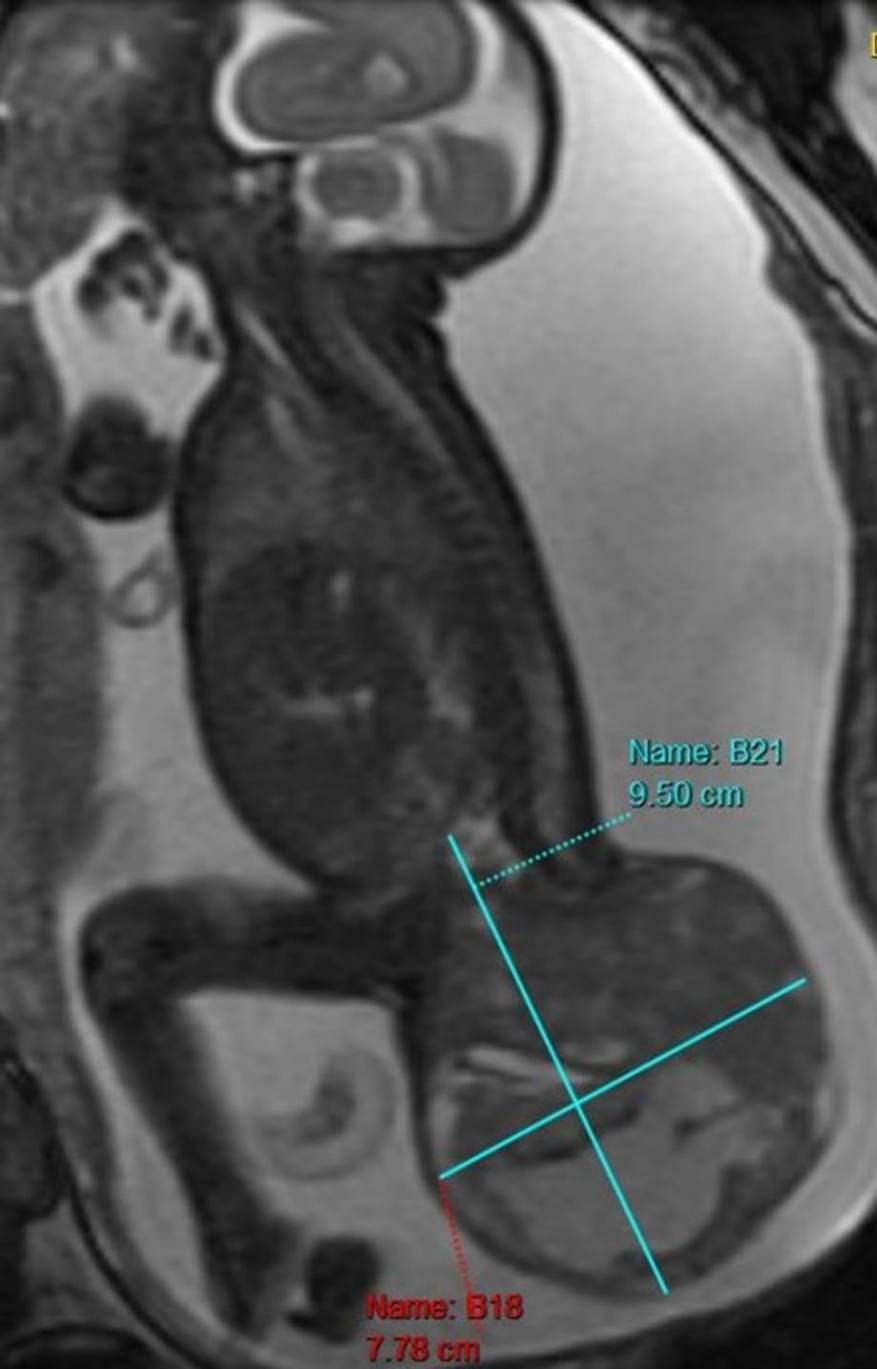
Fetal magnetic resonance image demonstrating a complex sacrococcygeal structure suspicious for a sacrococcygeal teratoma measuring 9.5 cm × 7.78 cm
